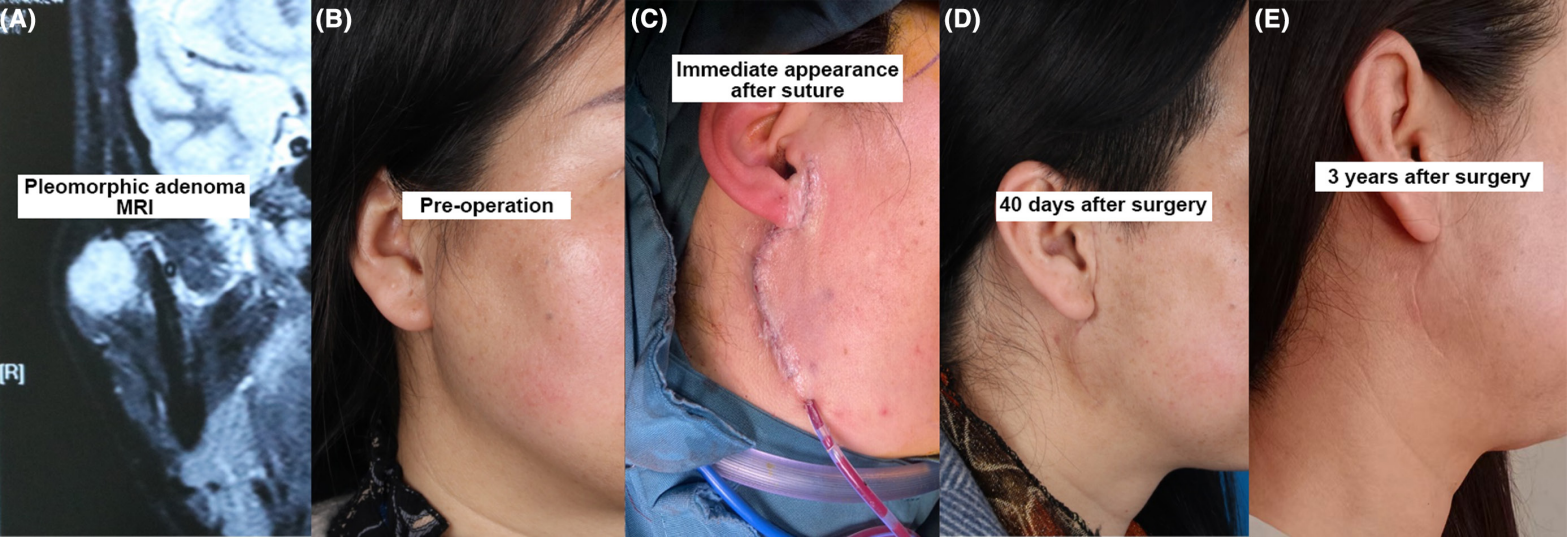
Preoperative and postoperative facial appearance of benign parotid tumors. (A) MRI image of pleomorphic adenoma. (B) Image of the patient's parotid region before surgery. (C) Immediate appearance after parotidectomy sutures. (D) Image of the parotid region 40 days after surgery. (E) Image of the parotid region 3 years after surgery.
